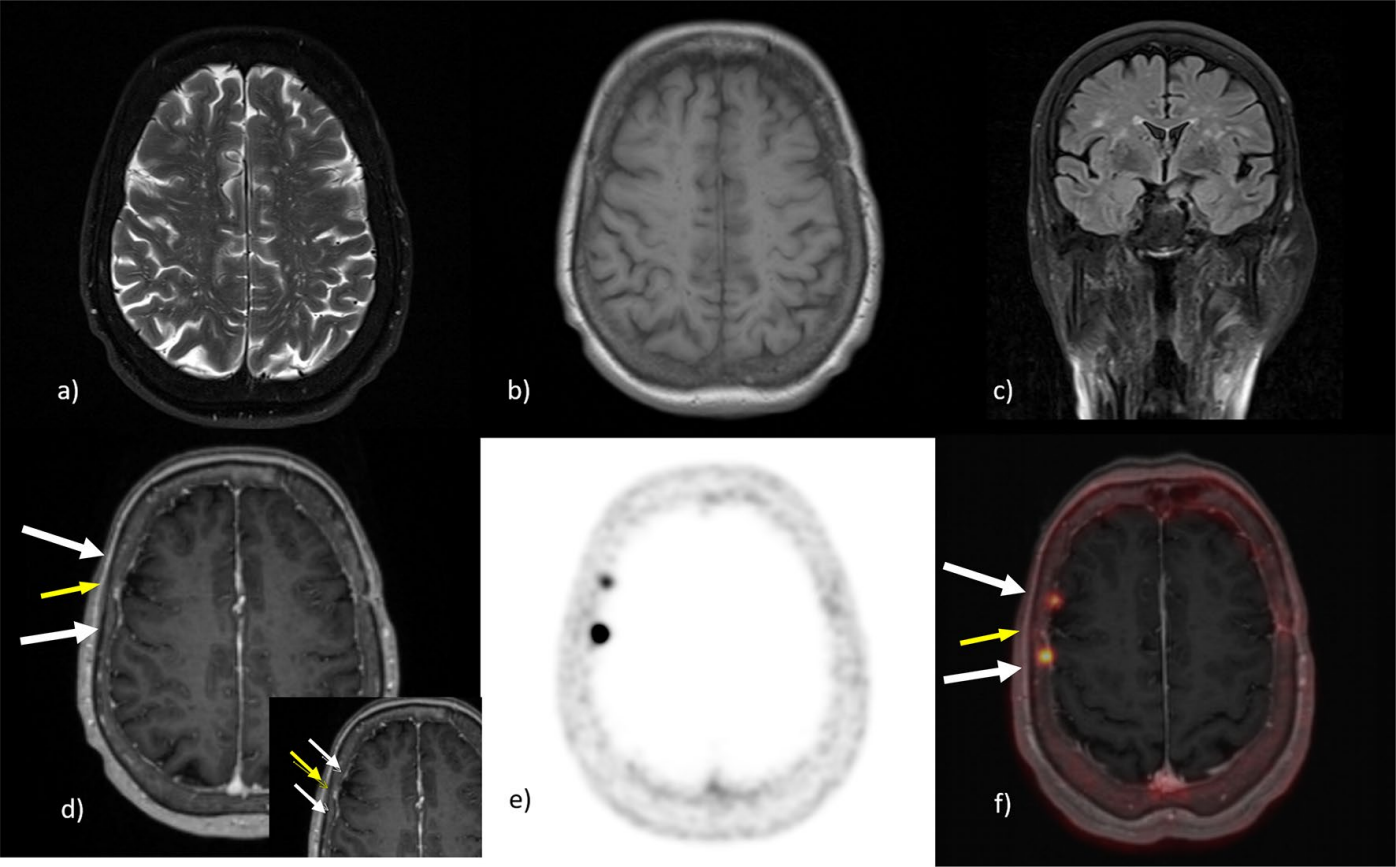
76 years old female patient with two small frontal ‘en plaque’ meningiomas (white arrows). Very close focus is necessary to distinguish the vessel in the center (yellow arrow) from the two surrounding meningiomas. The meningiomas were non-detected due to their small sizes (a) T2 TSE, (b) T1 FL2D and (c) TIRM. But they could have been detected in MPRage (d) the ‘en plaque’ meningiomas are clearly detectable in (e) PET und (f) PET/MRI fusion.
